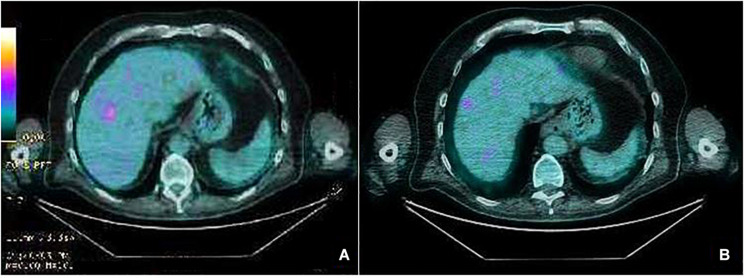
PET scan liver images before starting treatment with Pembrolizumab showing a pathologic standardized uptake value (SUV) (A). After 18 cycles of treatment, PET scan showing iRECIST complete response (B).
